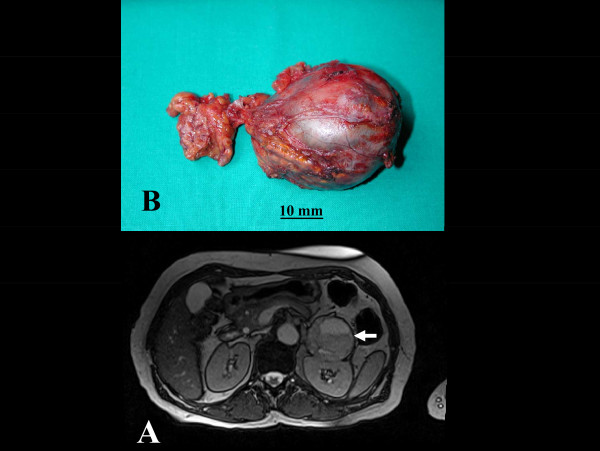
Pre-surgical MR and macroscopic anatomy images. A, abdomen MRI showing large left surrenal mass (white arrow); B, pheochromocytoma after surgical resection.
